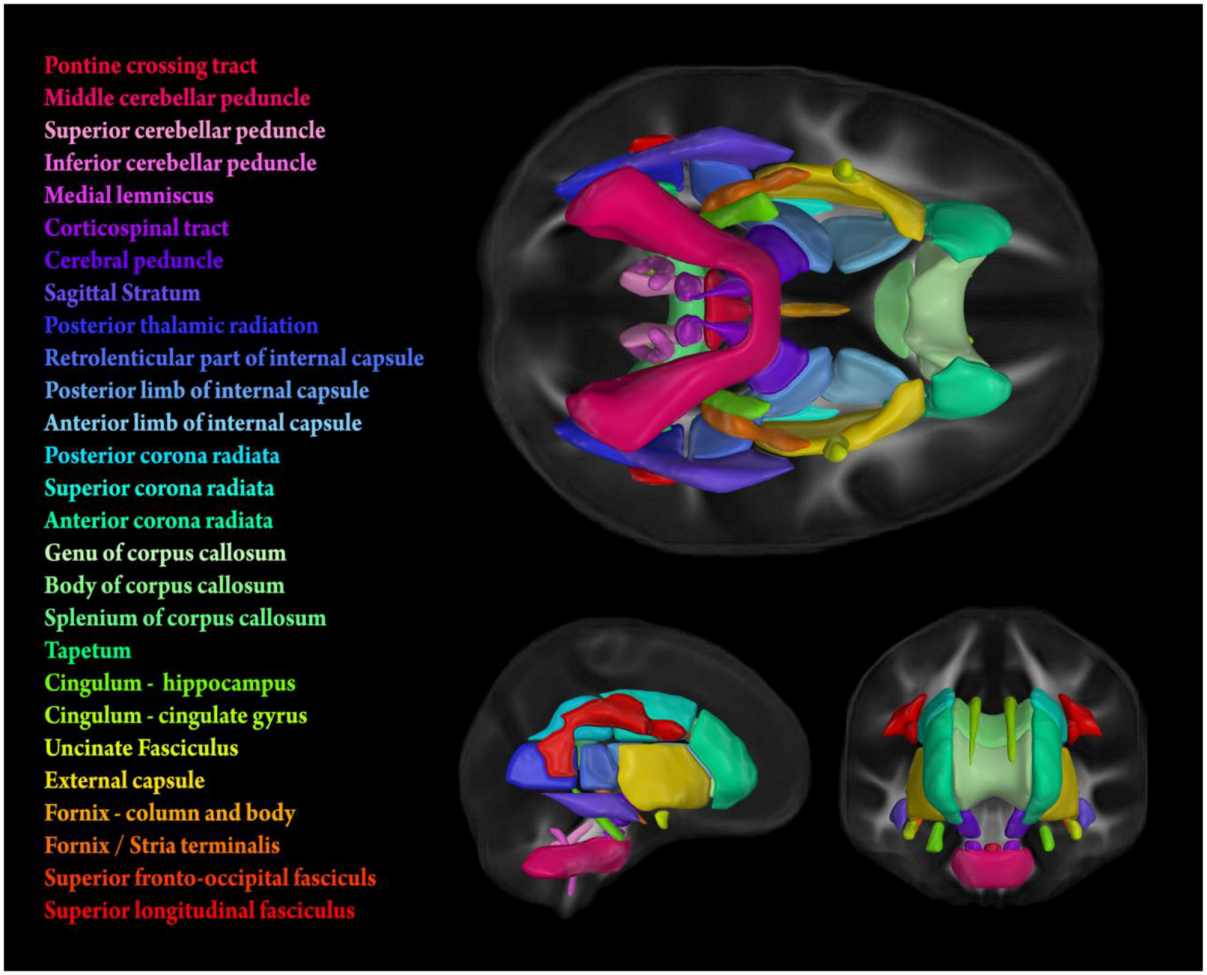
Stylized depiction of 21 bilateral and 6 midline tracts from the Johns Hopkins University (JHU) white matter parcellation atlas (Mori et al., 2005). FA values used for analyses were obtained by calculating tract-wise means from the intersection of the atlas and the individual FA skeletons generated using TBSS (see subsection MRI Data Acquisition and Processing for details).
